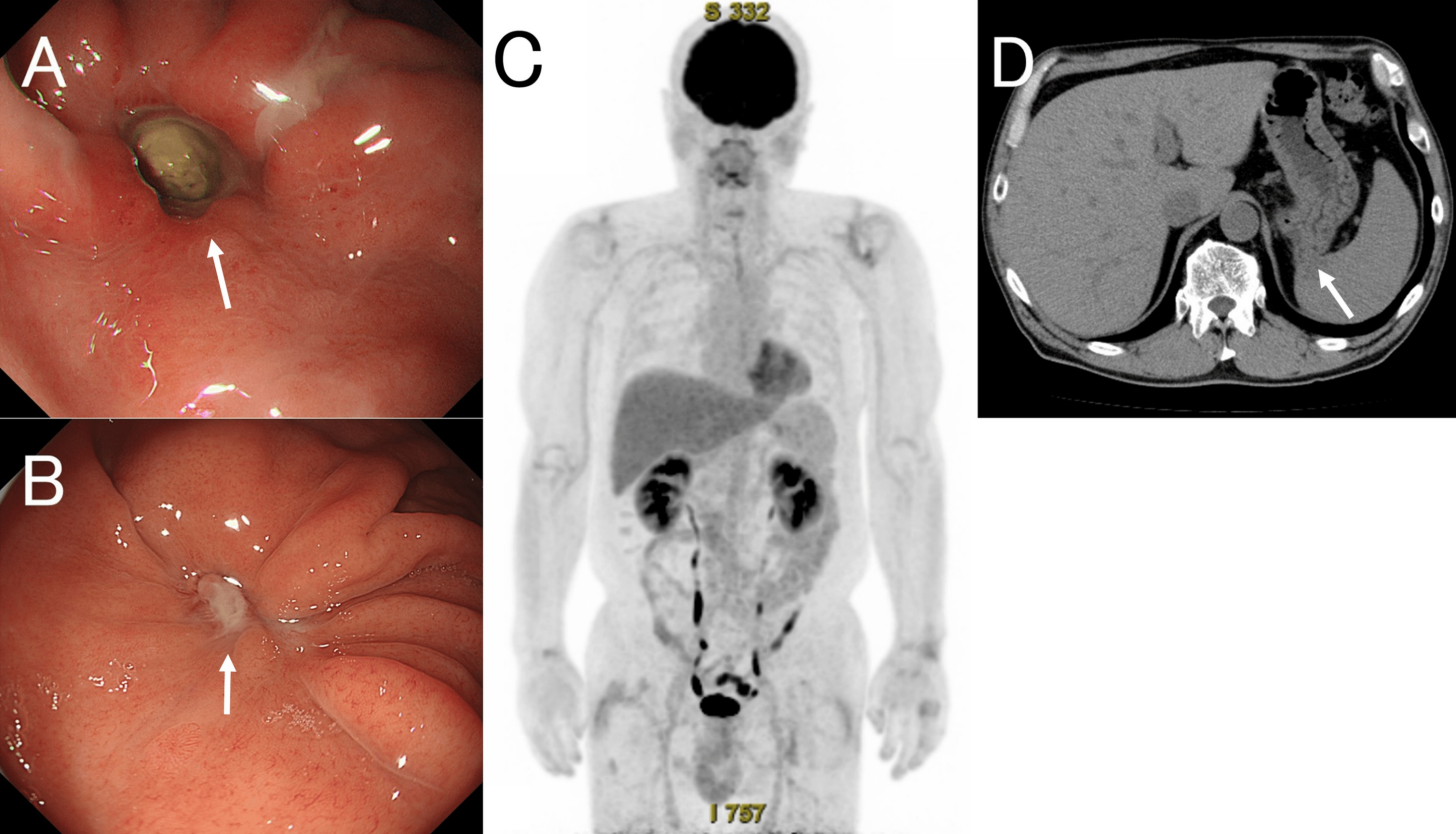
EGD and PET‐CT Images. (A) Esophagogastroduodenoscopy (EGD) after two cycles of chemotherapy shows the persistent fistula opening (white arrow). (B) EGD, performed six months after the initiation of chemotherapy, shows closure of the fistula (white arrow). (C, D) Positron emission tomography-computed tomography (PET-CT), performed seven months after the initiation of chemotherapy, shows no abnormal fludeoxyglucose F18 (FDG) uptake, consistent with complete metabolic remission. However, anatomical continuity between the stomach and spleen remains on CT (white arrow).
